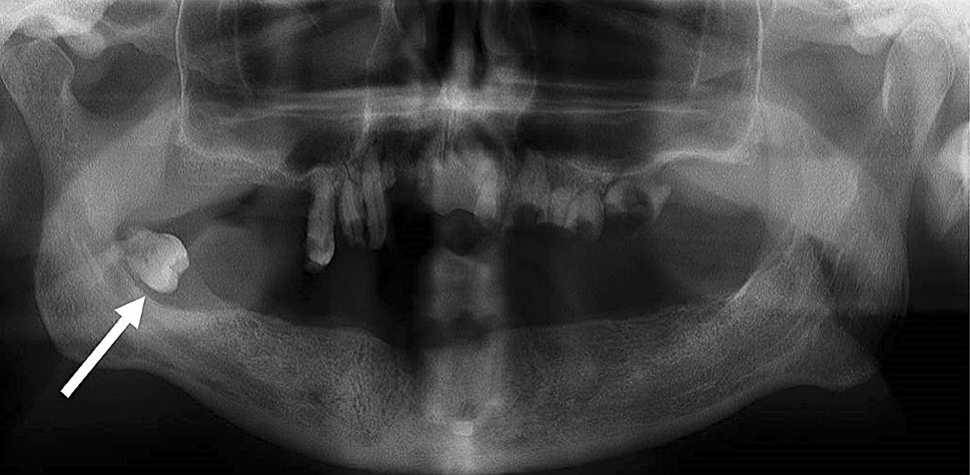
Orthopantomography showing dental foci and a wide radiolucency around the unerupted D48 (arrow)
